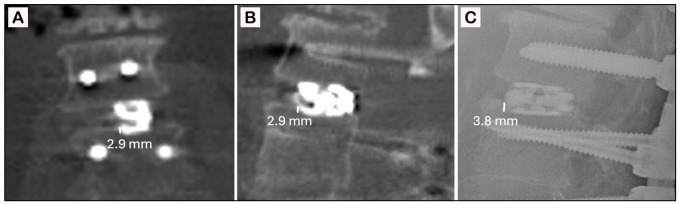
Modality-Dependent Variation in Interbody Cage Measurement After Lumbar Fusion.* (A) Coronal and (B) sagittal reformatted CT images, and (C) immediate postoperative lateral radiograph of the same patient after instrumented lumbar interbody fusion. White calipers indicate the endplate–implant measurement used to estimate cage position/subsidence. The measured value differs between CT and X-ray due to modality and projection effects (slice selection, magnification, and patient positioning). *Abbreviations:* CT = computed tomography.
